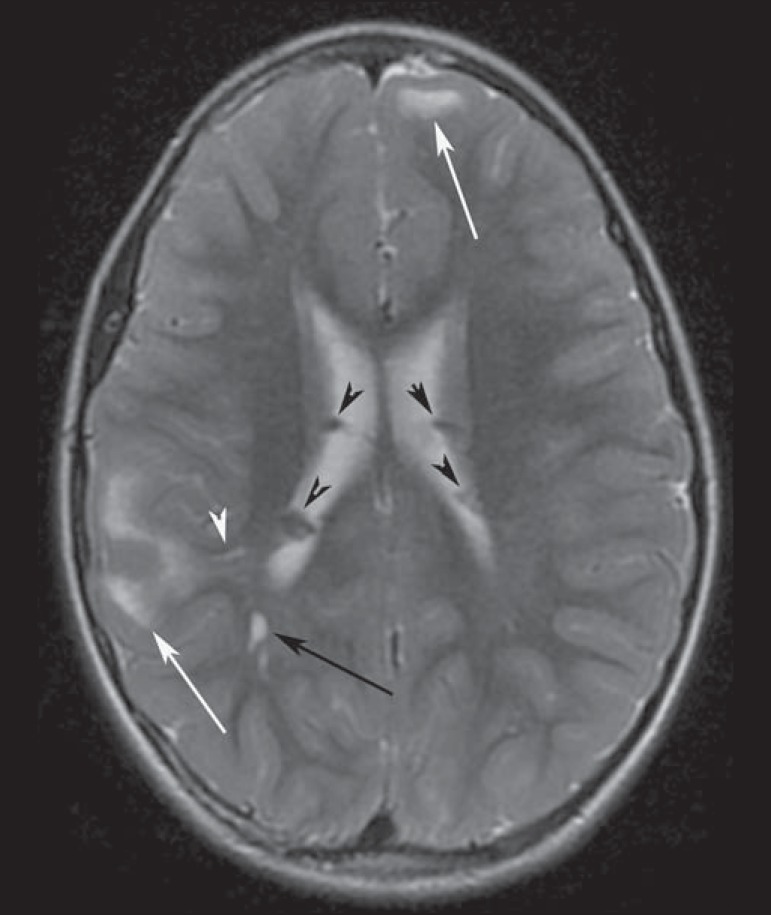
A 12-year-old boy with tuberous sclerosis complex. Axial T2-weighted
